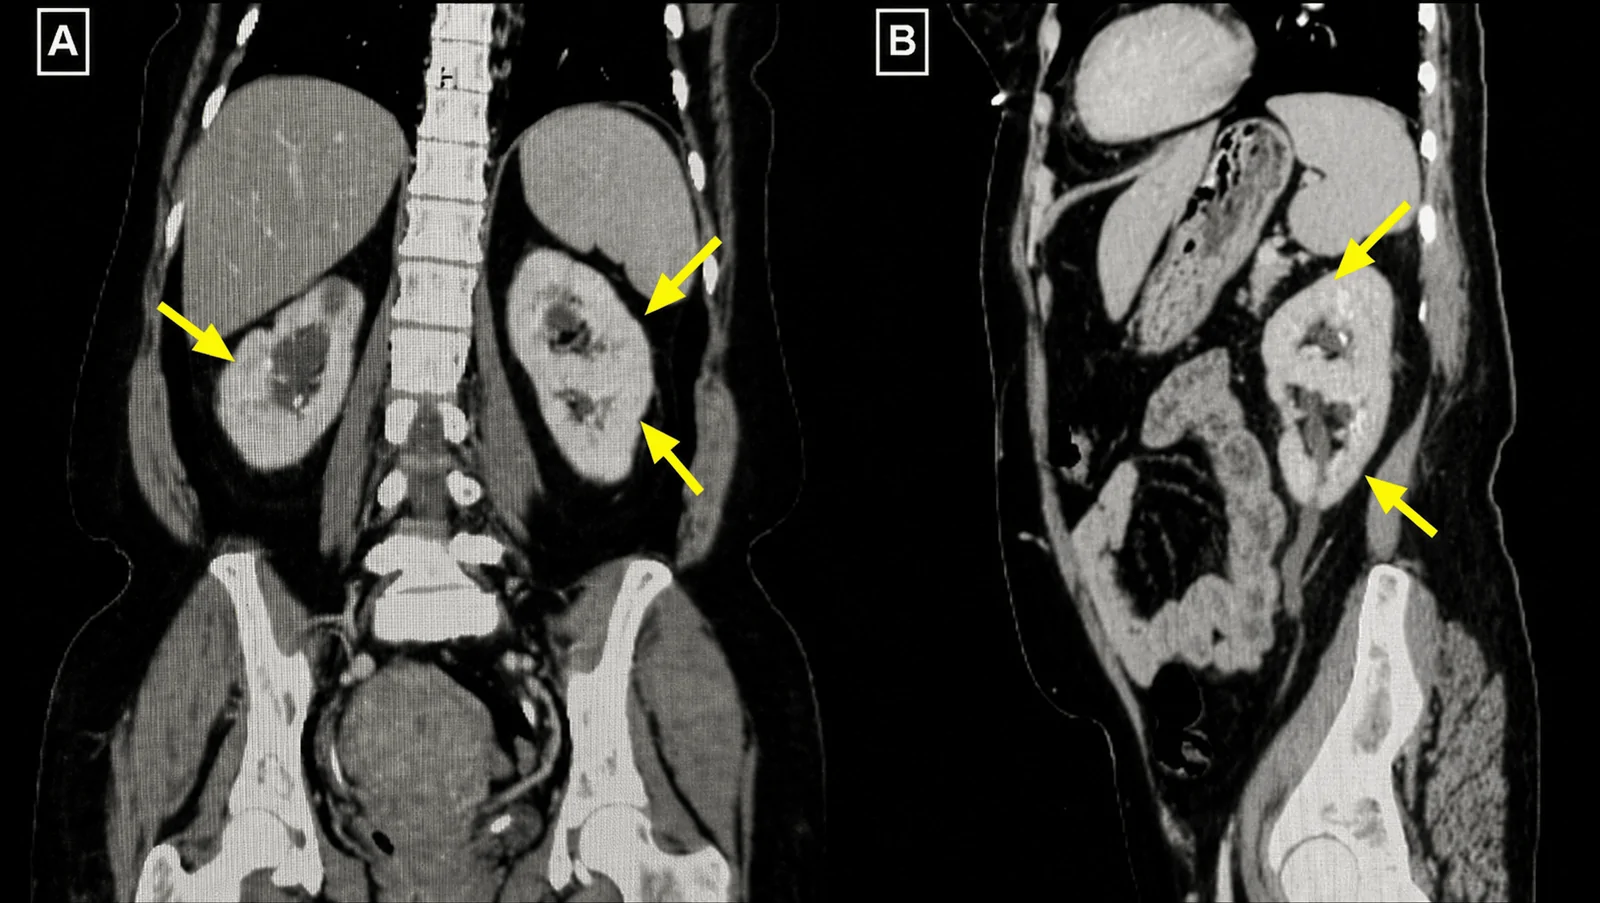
Contrast-enhanced CT of the abdomen and pelvis (A) Coronal image demonstrating bilateral medullary nephrocalcinosis with numerous calcifications concentrated in the renal pyramids, small non-obstructing renal calculi, mild left hydronephrosis, and partially visualized bilateral ureteral stents. (B) Sagittal image through the left kidney demonstrating mild pelvicalyceal dilatation and extensive medullary calcifications consistent with medullary sponge kidney. Bilateral ureteral wall thickening and periureteral fat stranding on the complete examination were consistent with ureteritis/pyelonephritis.
